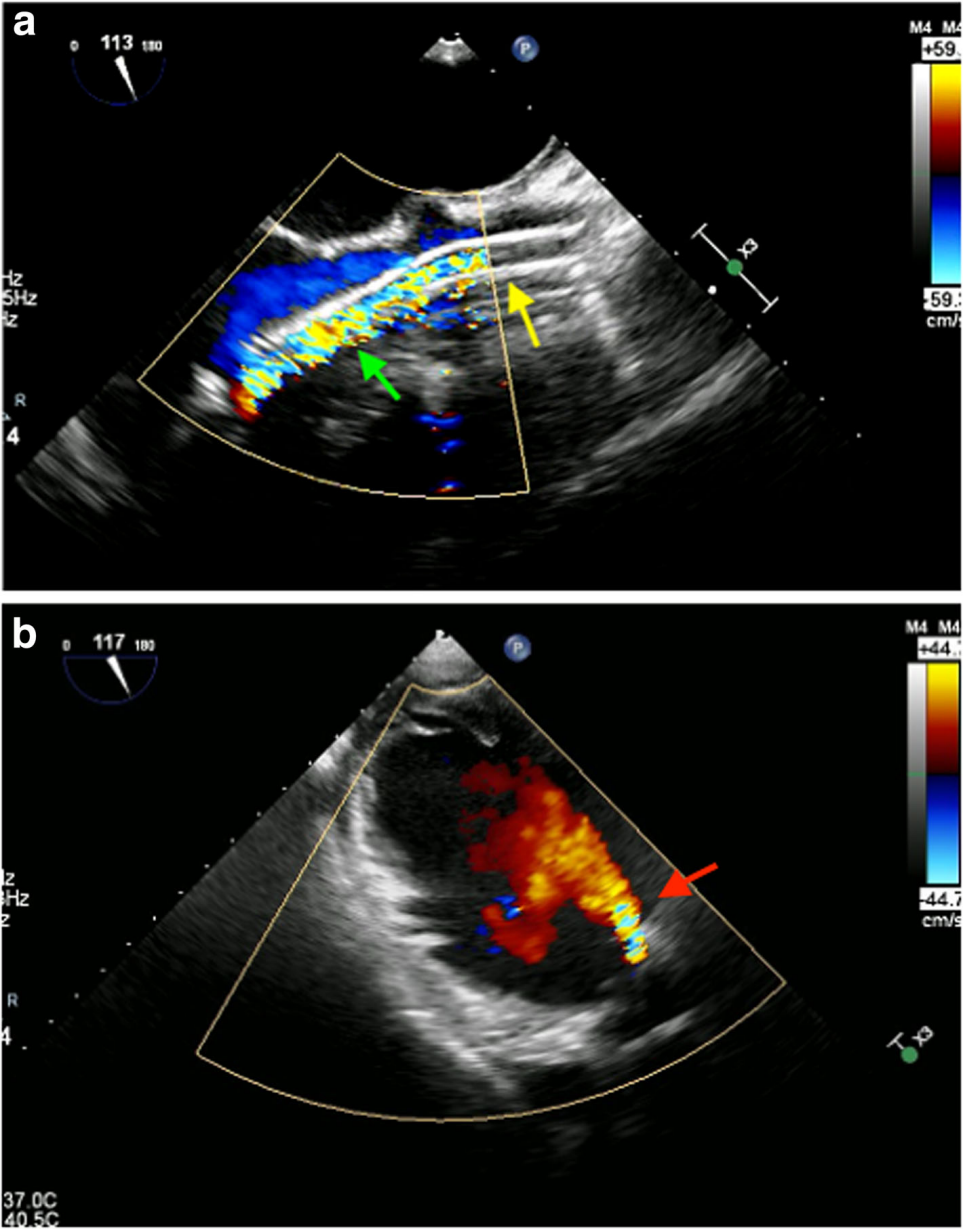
a Mid-esophageal long-axis view of transesophageal echocardiography. Yellow arrow indicates Impella cannula and green arrow indicates artifacts. Precise identification of aortic insufficiency by transesophageal echocardiography was difficult because of reverberation artifacts associated with axial blood flow inside of Impella. b Deep transgastric view of transesophageal echocardiography. Red arrow indicates regurgitation jet in the left ventricle due to aortic insufficiency. It was clearly identified without artifacts on this view
